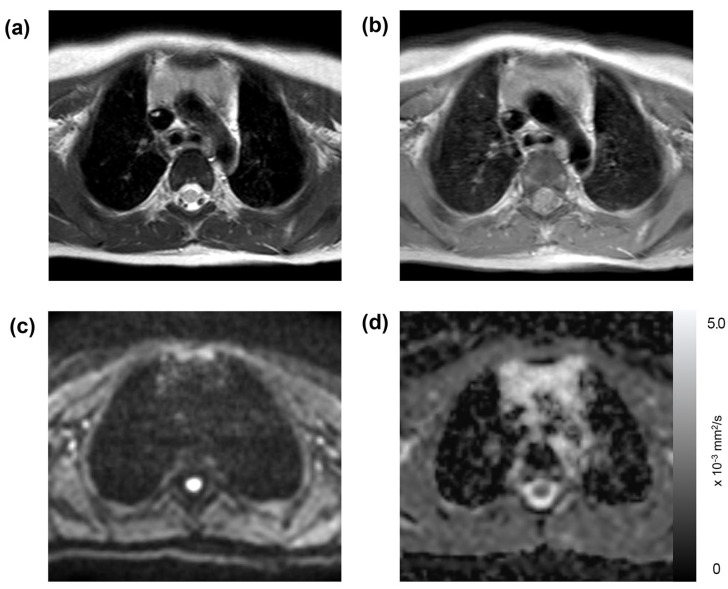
Unrestricted diffusion in the thymus: a 1-year-old boy with a history of mediastinal neuroblastoma. This patient underwent an MRI on a Philips scanner, with their laboratory data showing a WBC count of 1.22 × 10^3^/μL, an absolute neutrophil count of 0.67 × 10^3^/μL, an absolute lymphocyte count of 0.23 × 10^3^/μL, and a platelet count of 169 × 10^3^/μL. The thymus was found to be slightly hyperintense on both the T2-weighted (a) and T1-weighted images (b). A DWI with a b-value of 800 s/mm^2^ (c, “White dot” refers to a normal structure (normal spinal cord) that is unrelated to the lesion) exhibits hypointensity and no reduction in the ADC map with a mean ADC value of 1.96 × 10^−3^ mm^2^/s (d).
